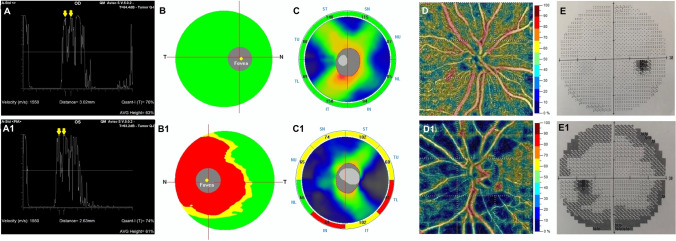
Right eye of a healthy subject (66-year-old female) shows normal values of optic nerve pial diameter (ONPD) at standardized A-scan ultrasound. The yellow arrows over the peaks of the two high and perpendicular spikes, as shown in echogram, indicate the maximum pial diameter measured in the anterior third of the optic nerve (A). Structural spectral domain (SD)–optical coherence tomography (OCT) revealed normal ganglion cell complex (GCC) (B) and retinal nerve fiber layer (RNFL) thicknesses (C). At OCT-Angiography (OCTA), the vessel density of radial peripapillary capillary (RPC) plexus does not show reduction (D), as well as there is no visual field loss (E). Left eye of a patient (68-year-old female) with primary open angle glaucoma reveals a reduction of ONPD at standardized A-scan ultrasound examination (yellow arrows shown in A1). Structural SD-OCT (B1, C1) parameters show a widespread damage at GCC and RNFL thicknesses. OCTA (D1) examination presents significant impairment in vessel density of RPC plexus. Peripheral visual field loss is present with respect to healthy subject (E1)
